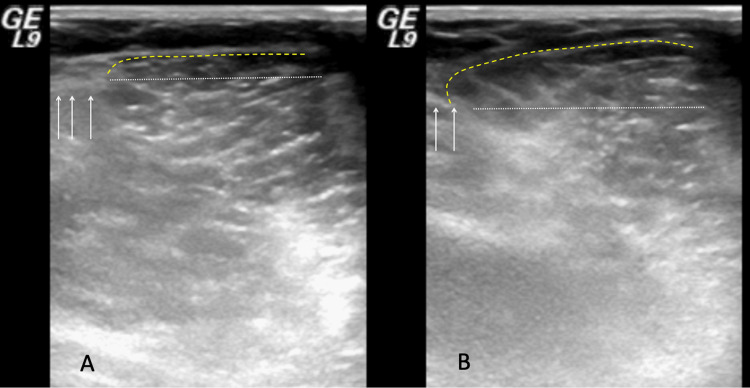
Ultrasonographic evaluation of the fascia defect both in relaxation (A) and contraction (B)
